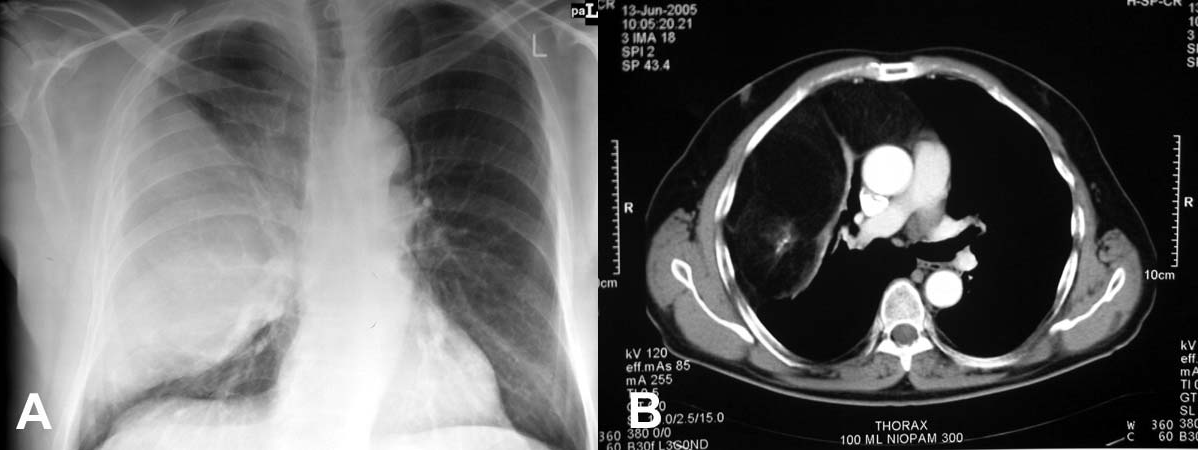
Chest radiograph showing soft tissue mass within the right hemithorax (A). CT (B) demonstrates that the mass contains low attenuation soft tissue suggestive of fat, and calcification, features in keeping with a teratoma.
